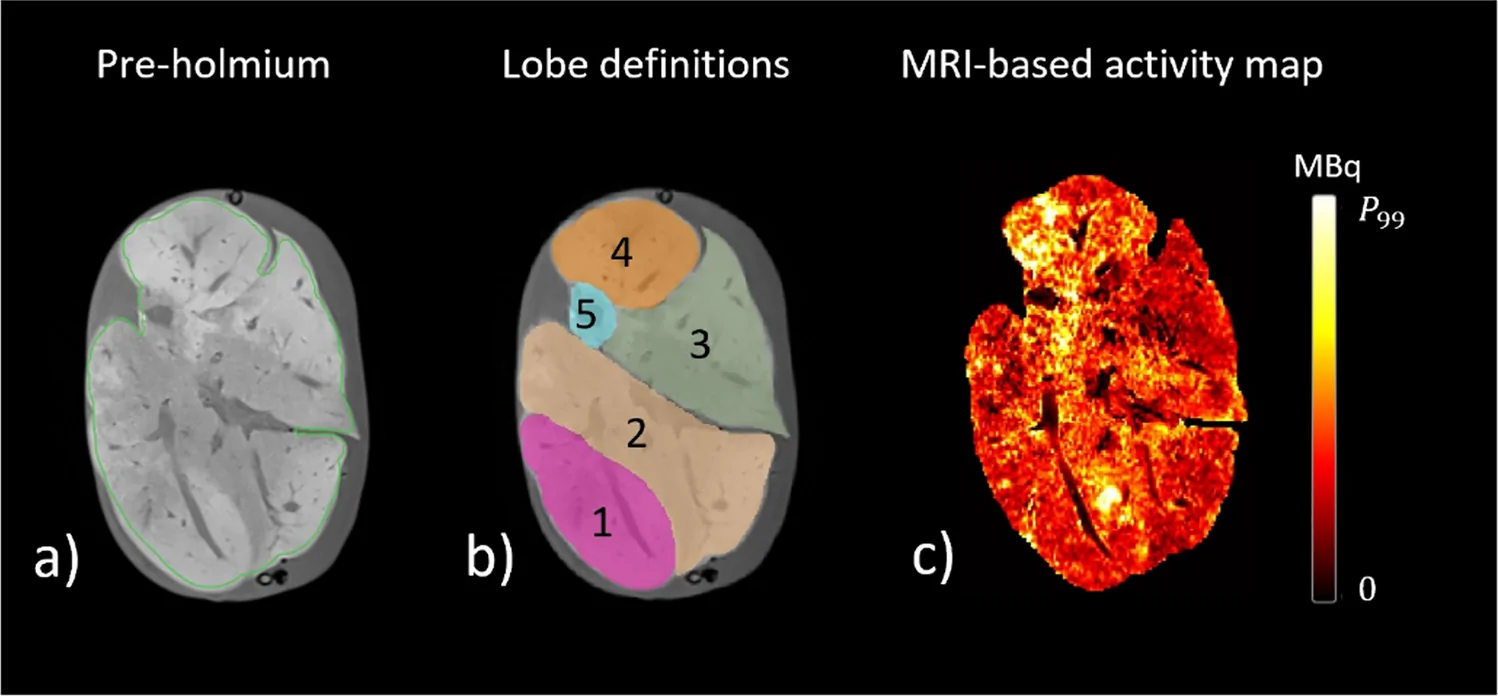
a Anatomical MRI scan of the ex vivo liver, b Lobe definitions, and c MRI-based activity map after administering 1250 mg of microspheres. The activity map was scaled to a maximum corresponding to the 99th percentile value
